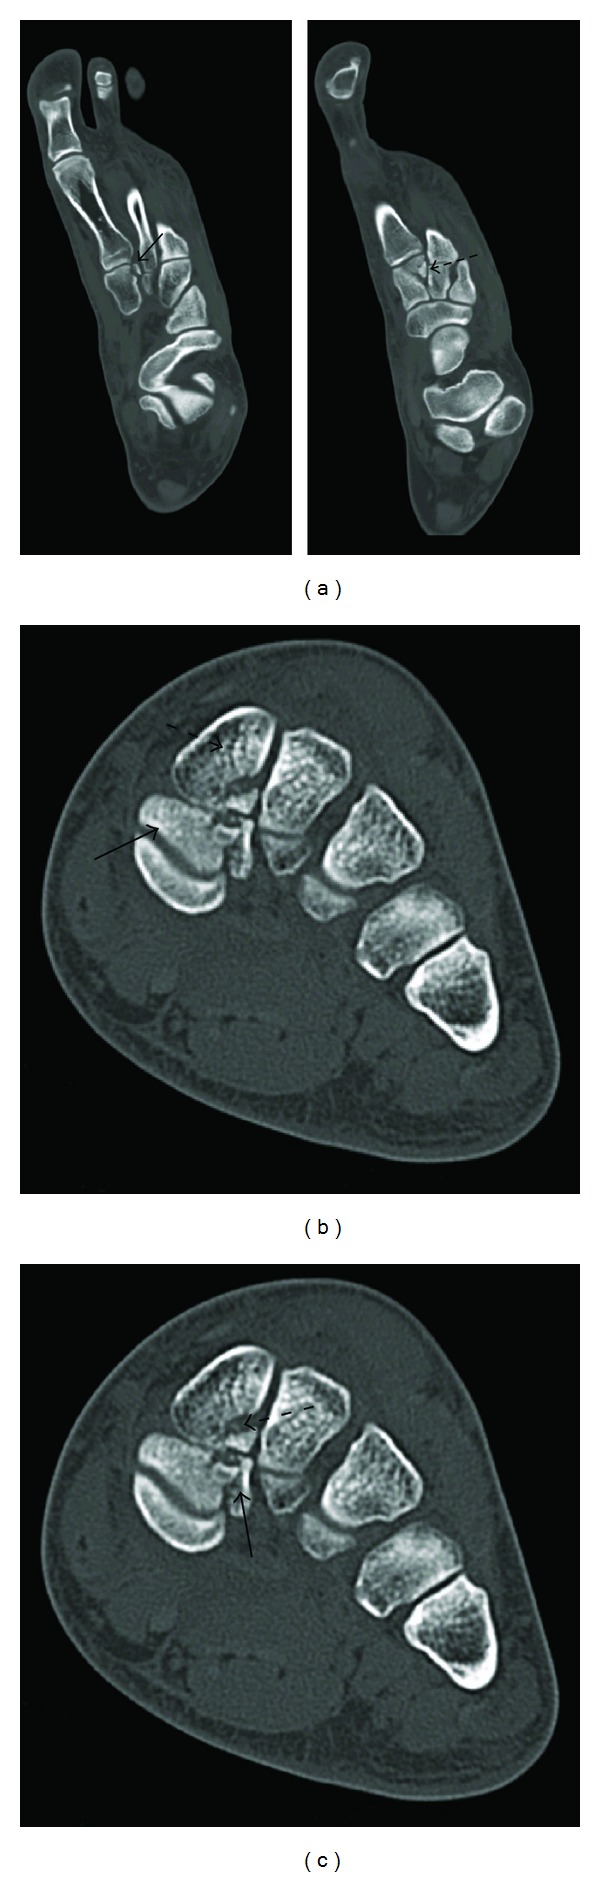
(a) Axial CT images at the level of the midfoot show a Lisfranc fracture of the medial cuneiform. The attachment of the plantar Lisfranc ligament (solid arrow) and the attachment of the Lisfranc ligament proper (dashed arrow) are demonstrated. (b) Coronal CT image at the level of the midfoot demonstrating the plantar (solid arrow) and dorsal (dashed arrow) segments of the bipartite medial cuneiform. (c) Coronal CT image at the level of the midfoot shows a Lisfranc fracture of the medial cuneiform. The attachment of the plantar Lisfranc ligament (solid arrow) and the attachment of the Lisfranc ligament proper (dashed arrow) are demonstrated.
